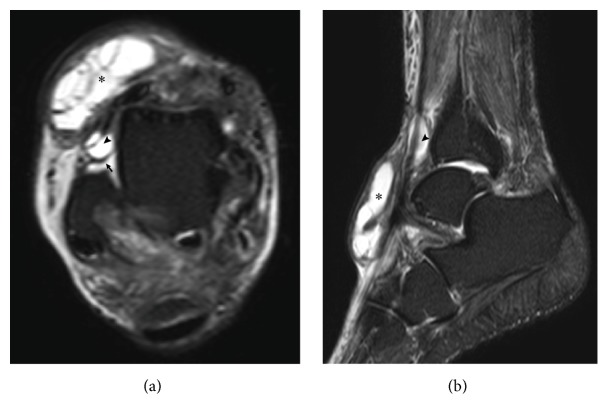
Magnetic resonance imaging of the right ankle. (a) An axial short T1 inversion recovery (STIR) image showing fluid collection in the lateral premalleolar bursa (asterisk). Fluid collection may also be seen in the tendon sheath of the extensor digitorum longus (EDL) (arrowhead). The anterior talofibular ligament is unstrained (arrow). (b) A sagittal STIR image showing fluid collection in the tendon sheath of the EDL just anterior to the ankle joint.
